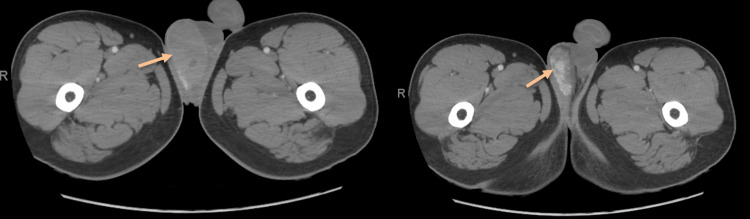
A pelvic CT (transverse view) revealing a heterogeneous enlarged right testes with central foci of hypodensity.
